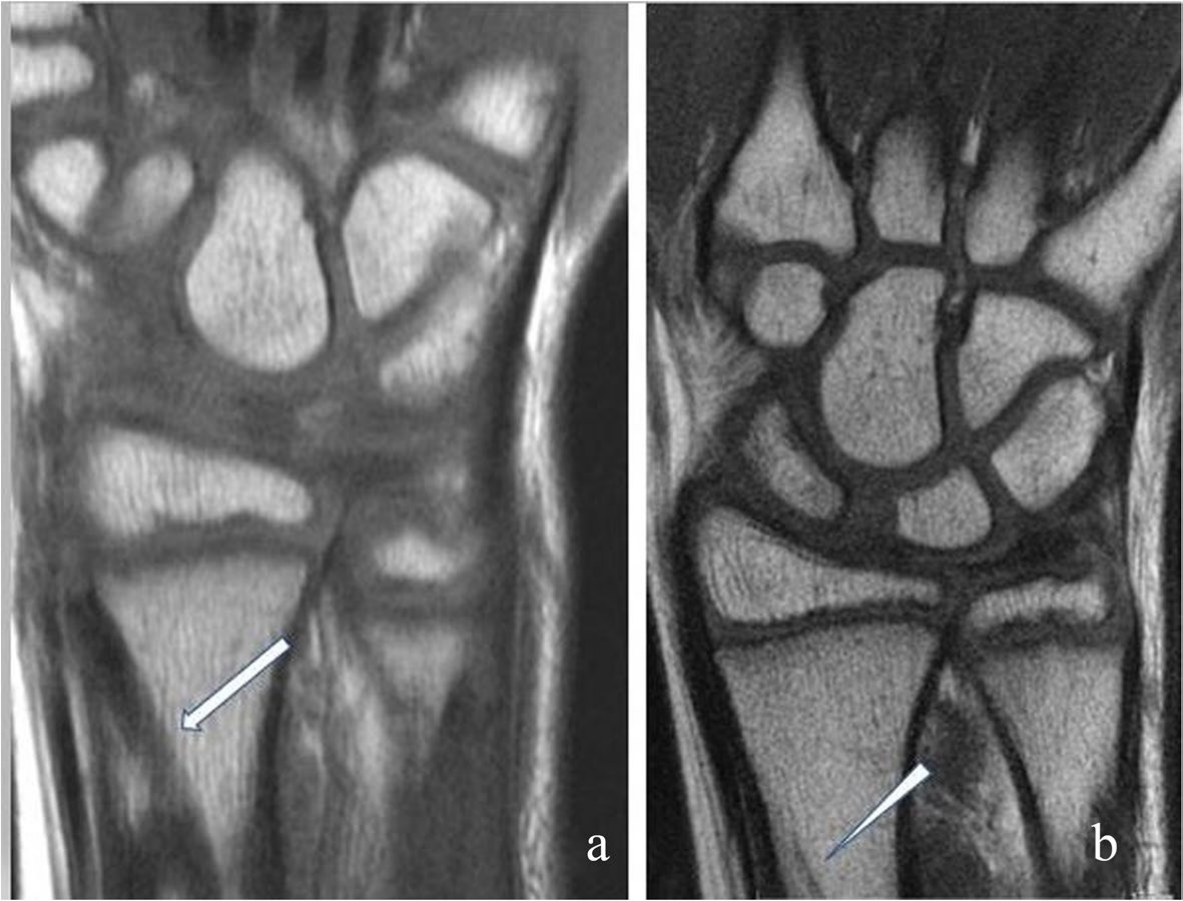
MRI image after PLGA implant placement a: half a year after the surgery, the implant is clearly visible (arrow) b: two years later only minimal traces of the implant are visible (arrowhead). There is no sign of growth disturbance
